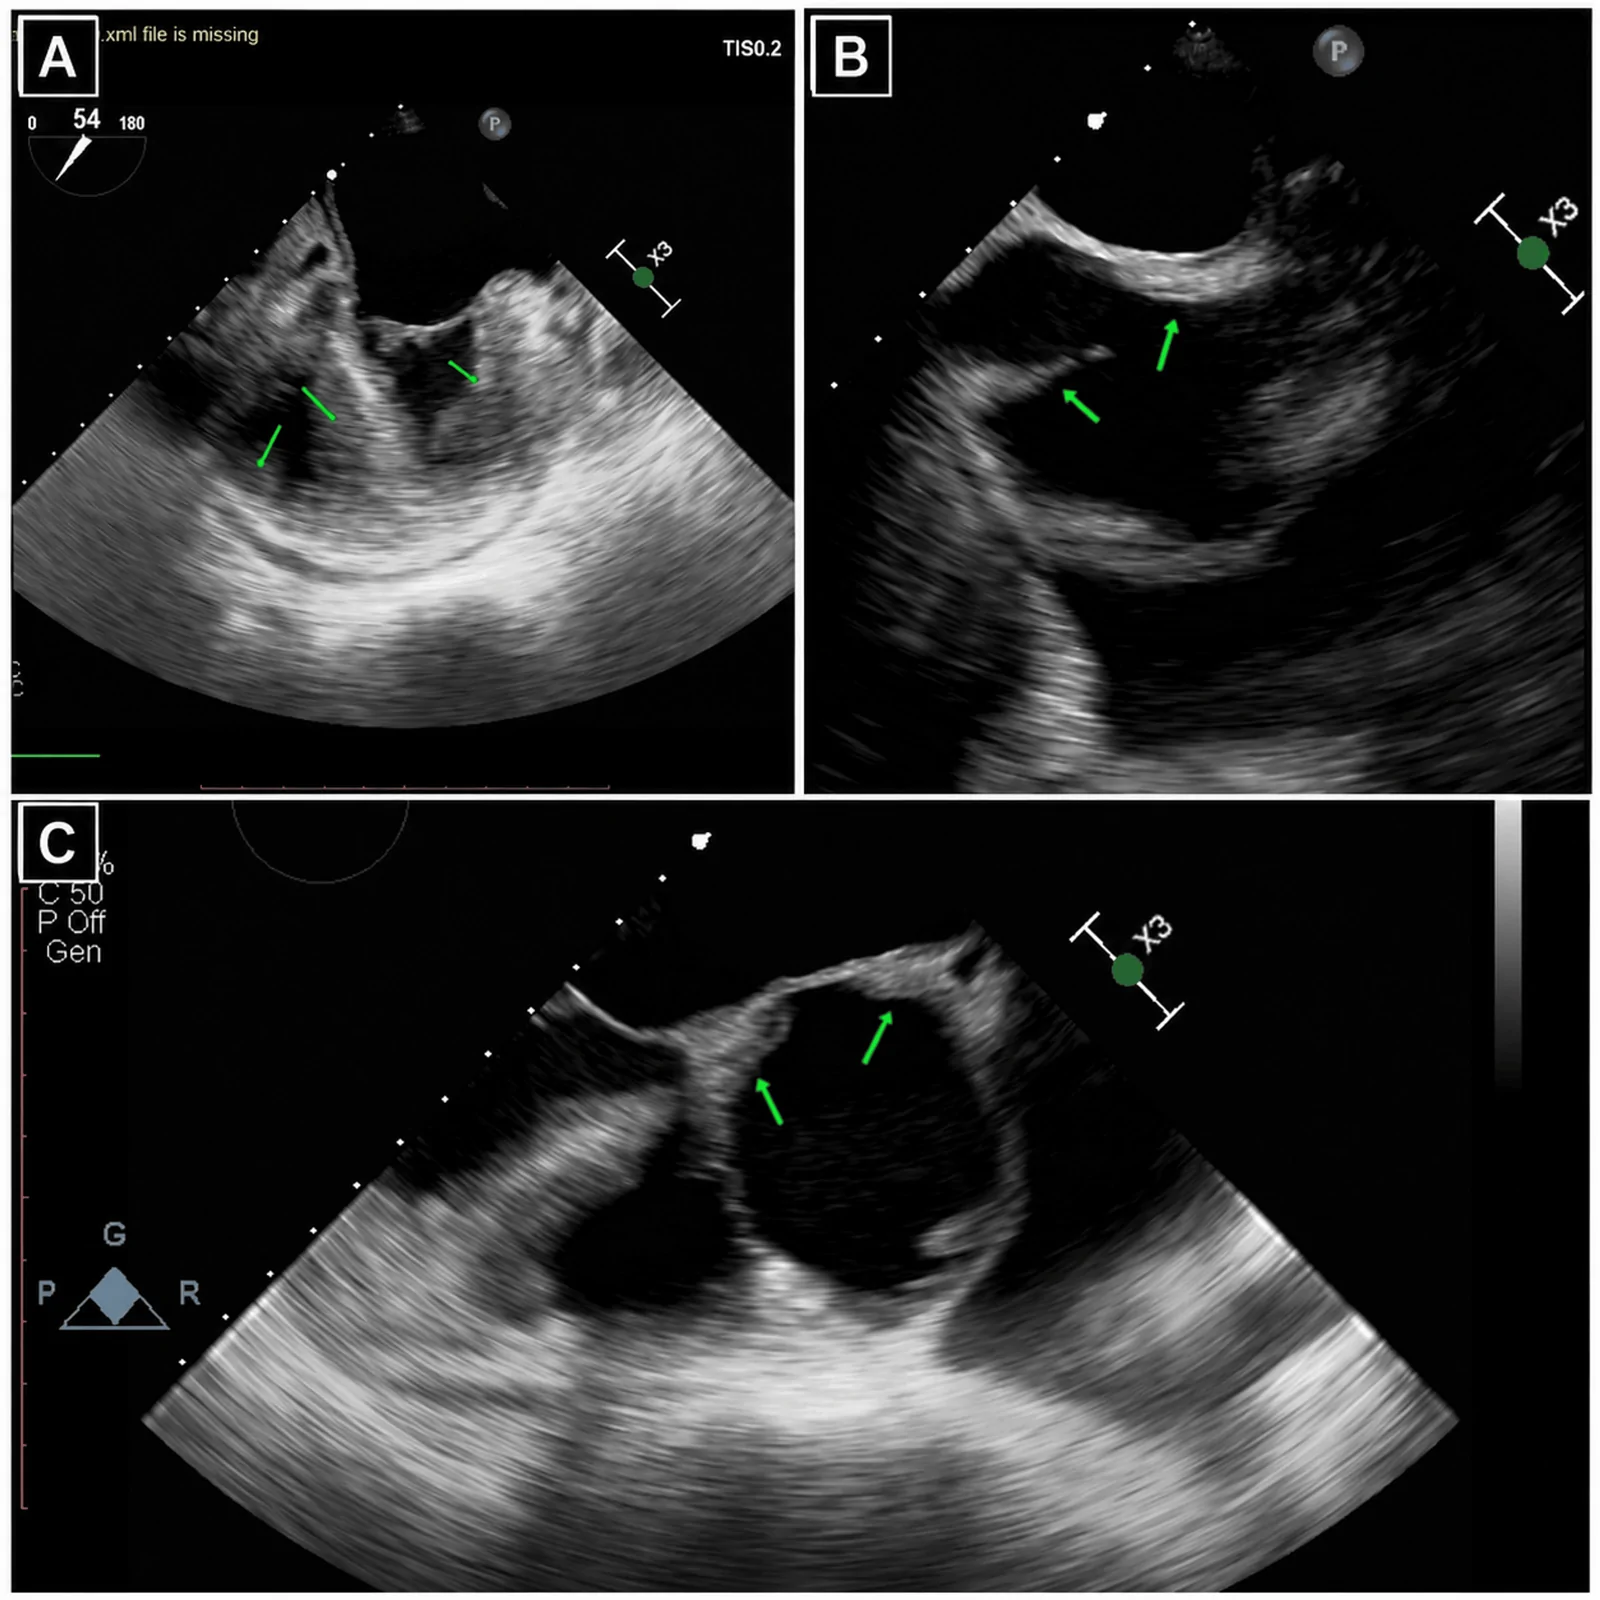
Transesophageal echocardiogram. A: Four-chamber view demonstrating biventricular hypertrophy. B: Bicaval view demonstrating left atrial wall thickening suggestive of infiltrative involvement. C: Four-chamber view also demonstrating left atrial wall thickening suggestive of infiltrative involvement
